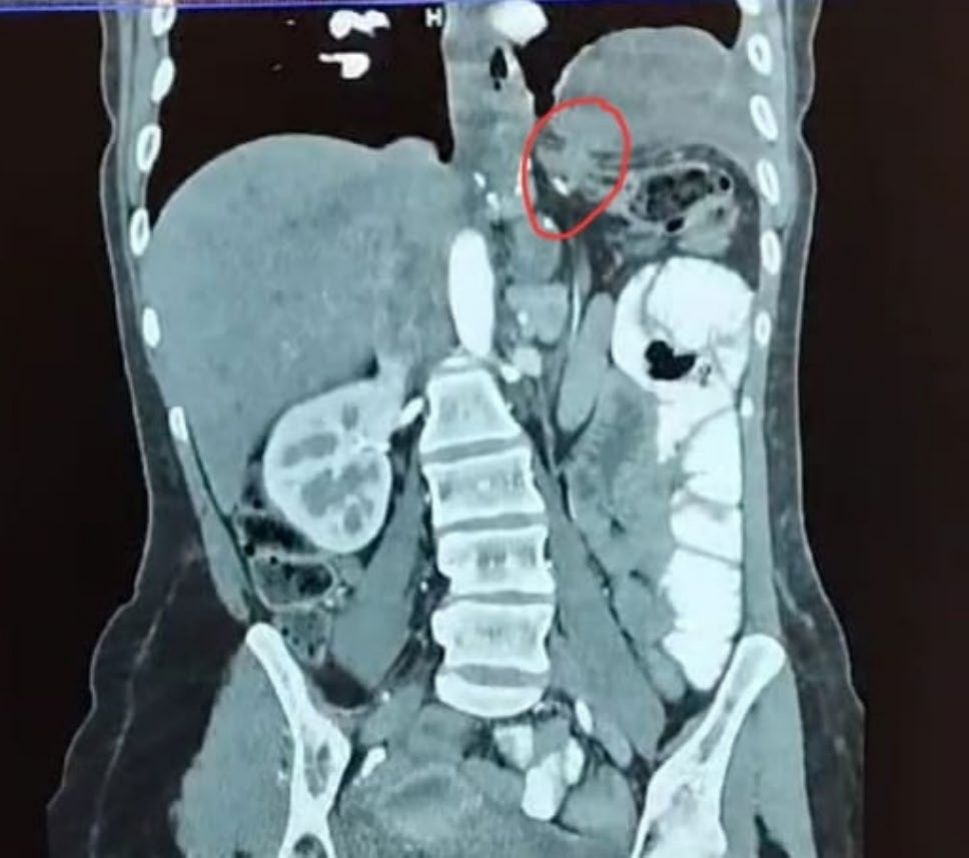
CT chest and abdomen showing herniation of a hollow organ through a small diaphragmatic opening
